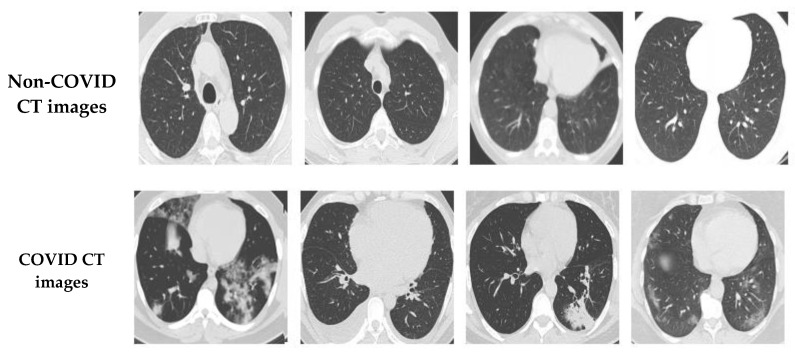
Sample COVID-19 and non-COVID CT images.
